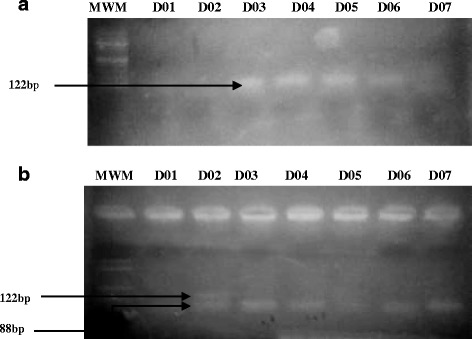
RFLP-PCR of ABCC8 C49620T Polymorphism. a shows the presence of the amplified gene for D01-D07 at 122 bp. b shows the restriction digest products of the gene. A 122 bp indicates the T allele while a 88 bp indicates the C allele. Sample D01 and D03-D07 show the presence of the wild-type homozygote CC genotype while D02 shows the presence of the heterozygote CT genotype. MWM indicates 100 bp molecular weight marker
